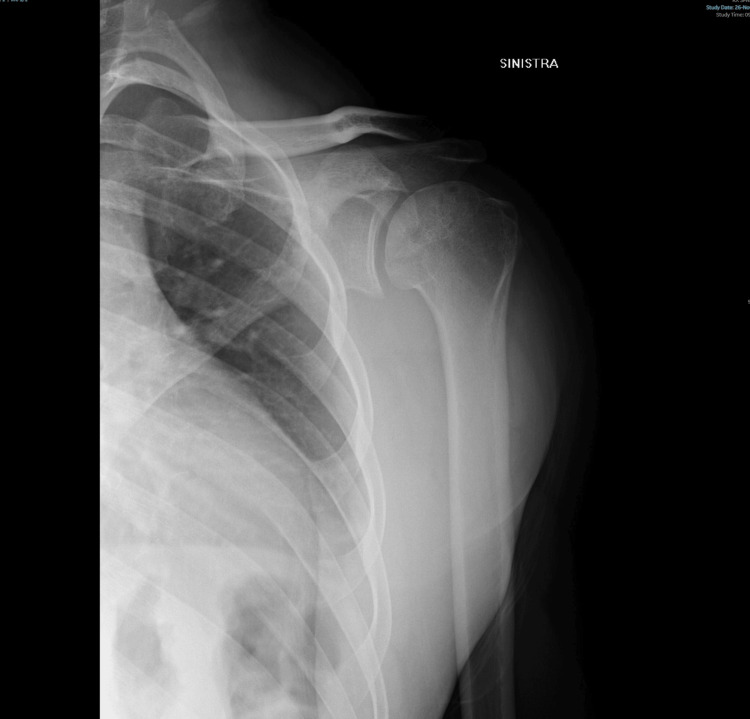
Immediate postoperative anteroposterior radiograph.
